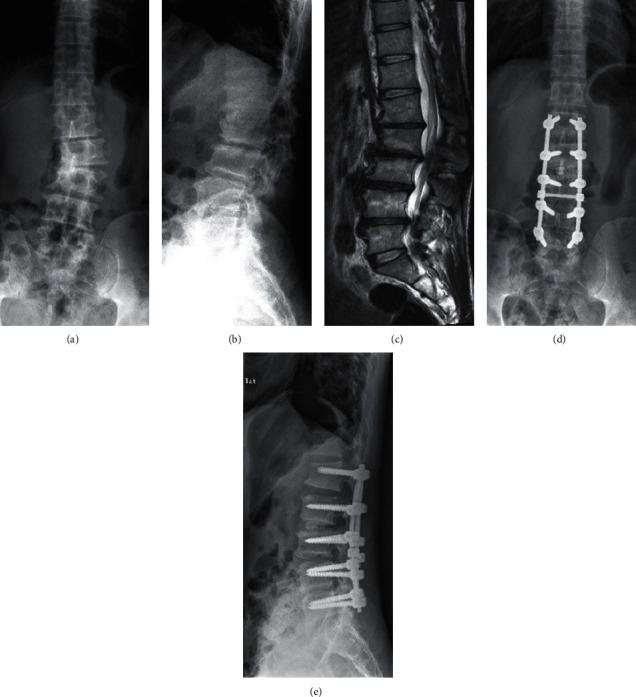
A 46-year-old man had spinal stenosis at L1–5 with DLS (a–c). She underwent fenestration decompression at L4-5, TLIF at L2-3, and posterolateral instrumented fusion at L1–5. The radiographs obtained 38 months after the operation showed stable scoliosis correction (d, e).
